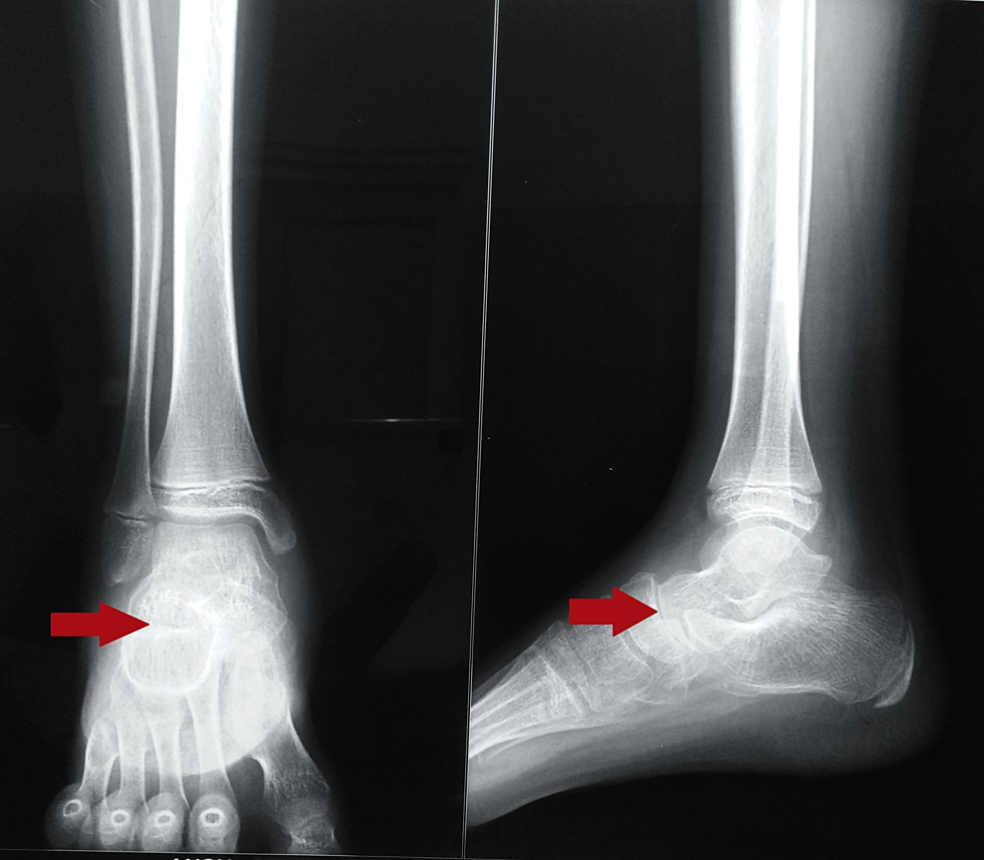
Radiograph of the right foot (anteroposterior and oblique views) showing gross talonavicular joint space narrowing
